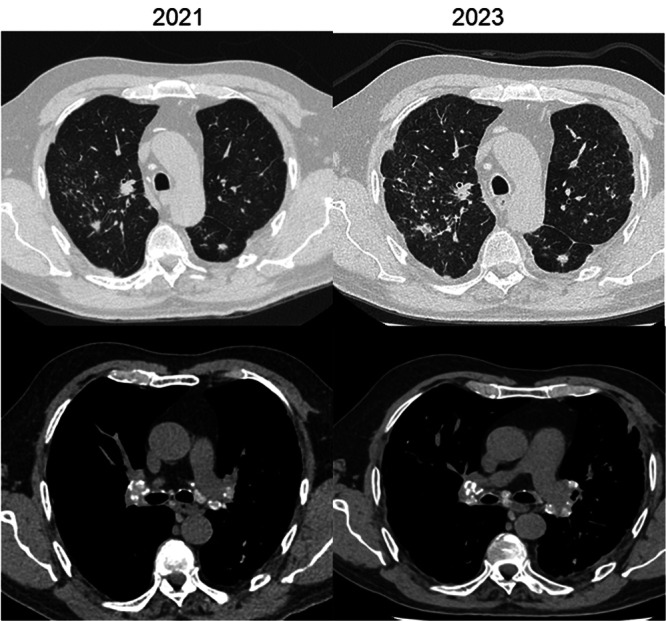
Silicosis (Cases 1, 5, and 11). Typical HRCT findings of *silicosis*, including upper‐lobe–predominant micronodules, pseudoplaques, and calcified hilar lymphadenopathy (“eggshell” pattern). Functional progression occurred despite radiological stability in two patients (Cases 1 and 5), emphasizing that physiologic decline may precede imaging deterioration.
